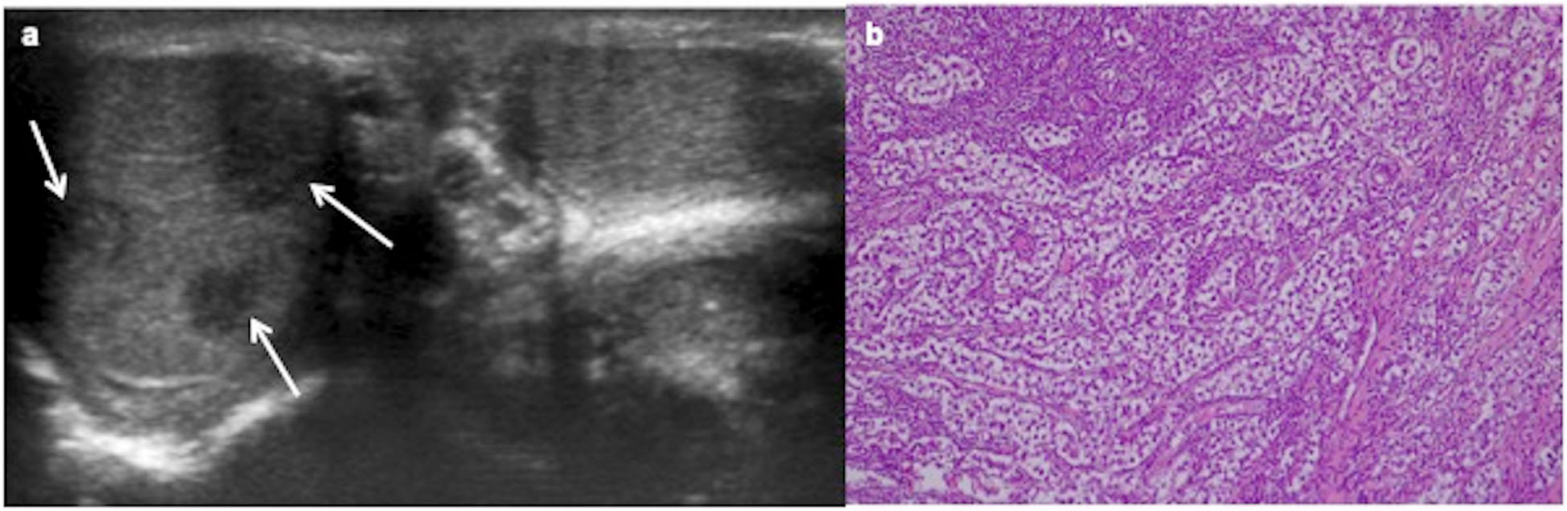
Seminoma. A 13-year-old boy with a right testicular mass since two months ago. a Transverse ultrasound scan of both testes shows an enlarged right testis with multinodular hypoechoic mass (arrows). Intraoperative biopsy diagnoses seminoma and an inguinal orchiectomy is practised. b Sheets of relatively tumour cells within fibrous bands and lymphocytic infiltrate
